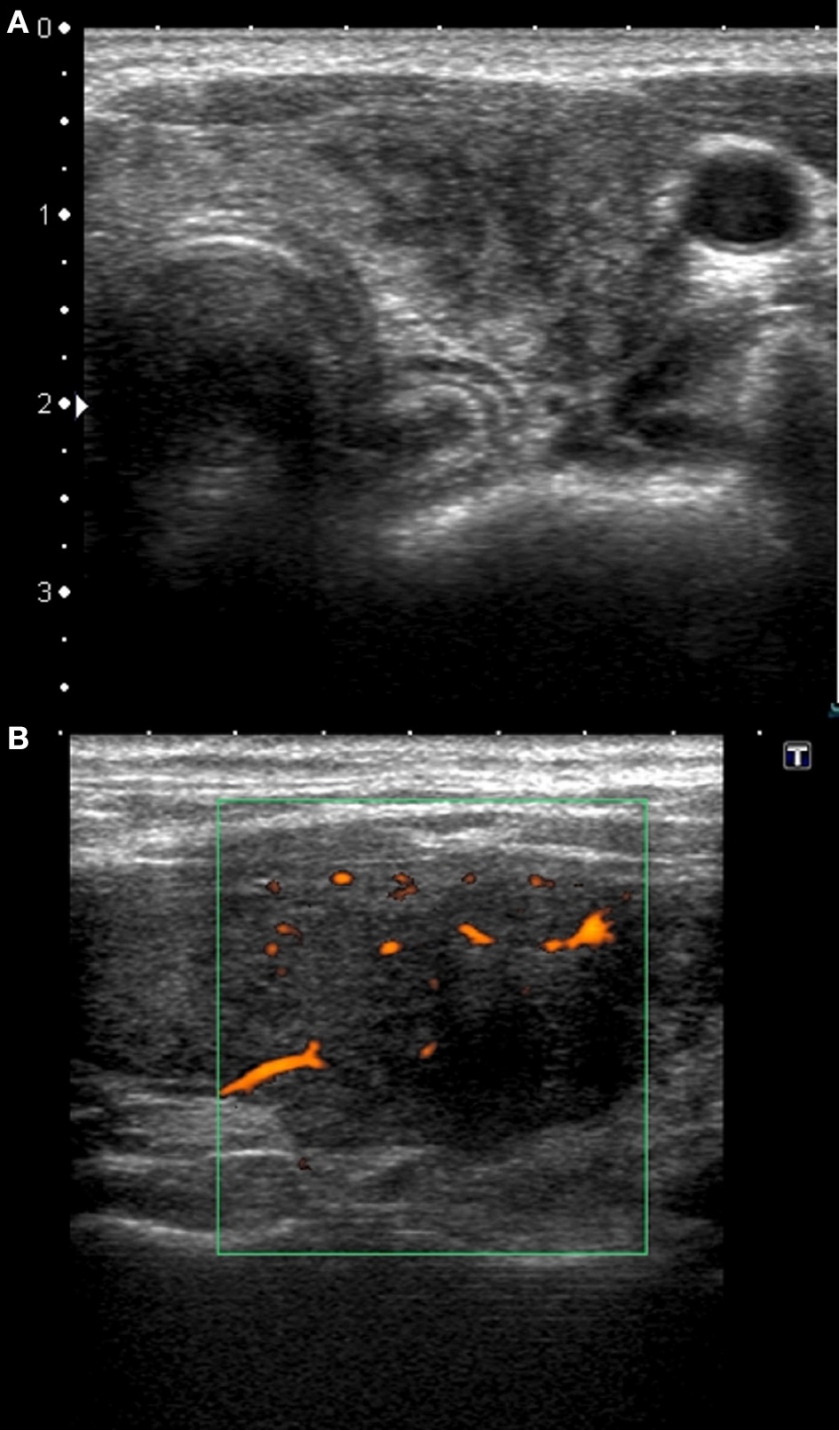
Typical sonographic patterns of SAT in patients with HLA-B*35, C*04:01, and DRB1*01, without HLA-B*18:01. (A) Hypoechoic, strongly heterogeneous, areas with poorly defined margins; (B) hypoechoic blurred areas with decreased or absent vascularization.
